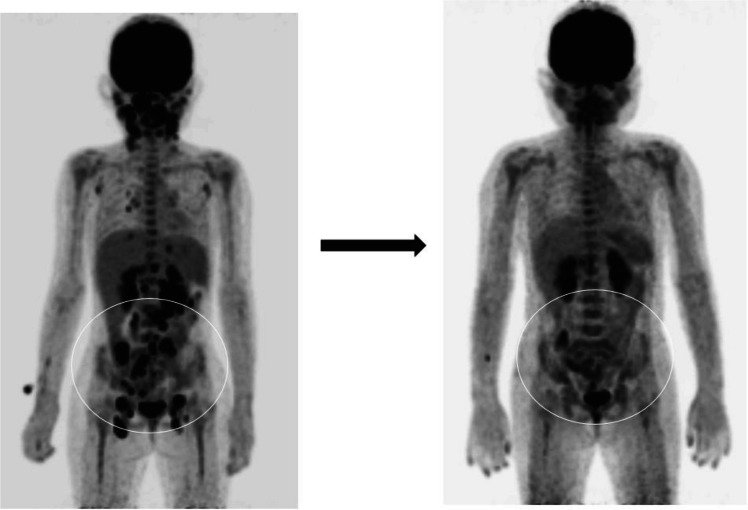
Representative PET–CT image showing increased fluorodeoxyglucose uptake in the small bowel. The lesion is indicated by a circle
